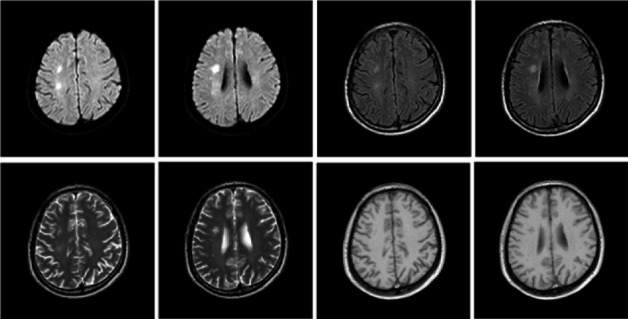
Head MRI (January 16, 2018 in Liaocheng People's Hospital) showed hyperintense lesions on DWI or hyperintense lesions on T2WI in the right semiovale center and radiating corona.
